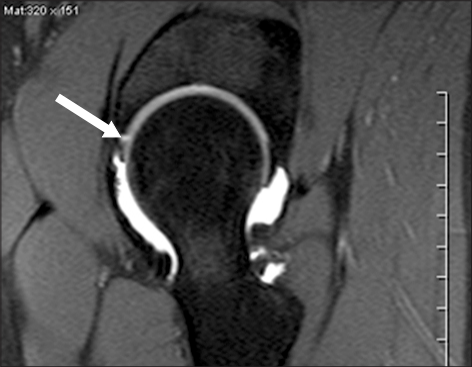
MR arthrogram in the T1-weighted image shows a labral tear at the anterosuperior portion.
